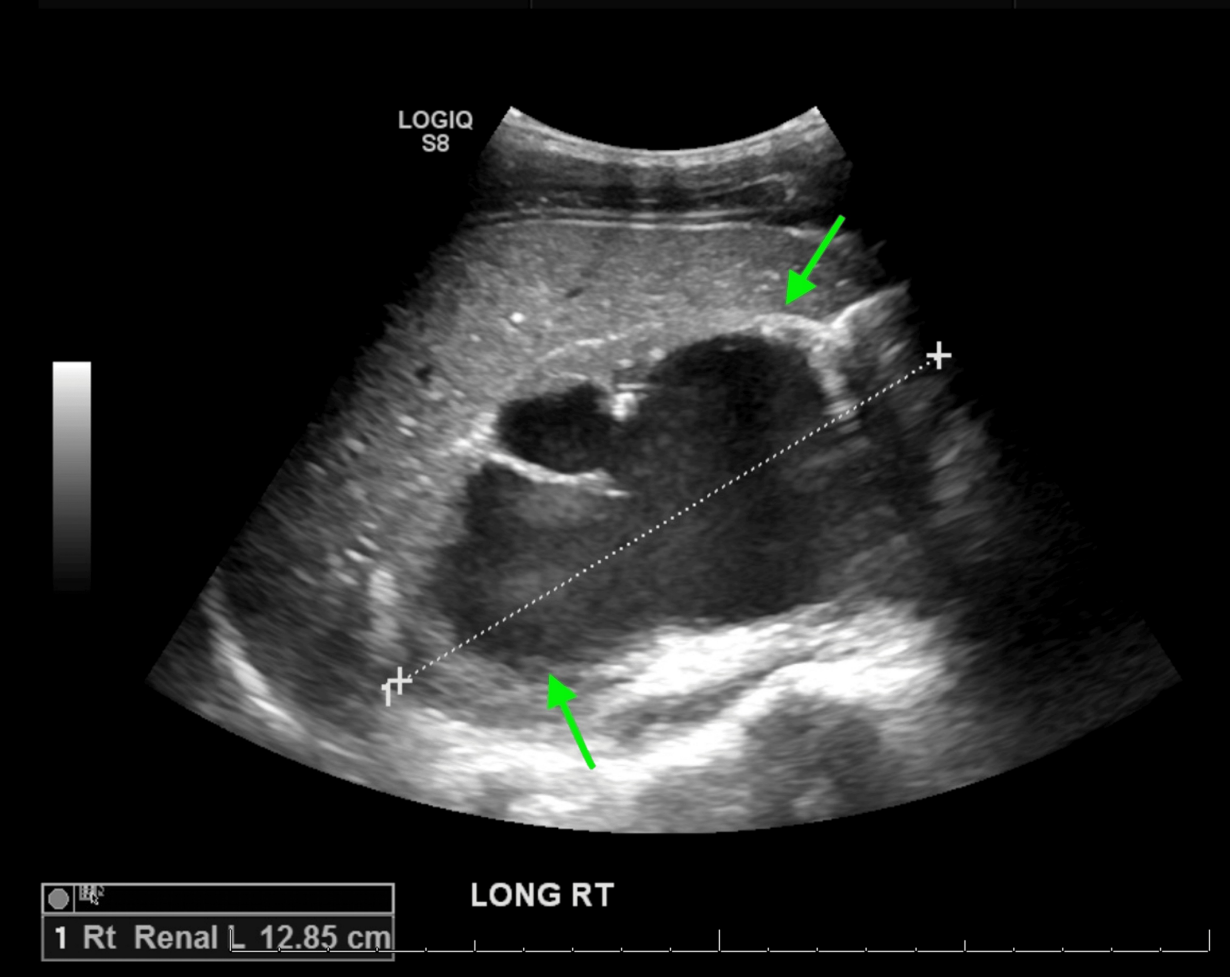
Right-sided renal ultrasound shows severe hydronephrosis similar to that on the left side.
